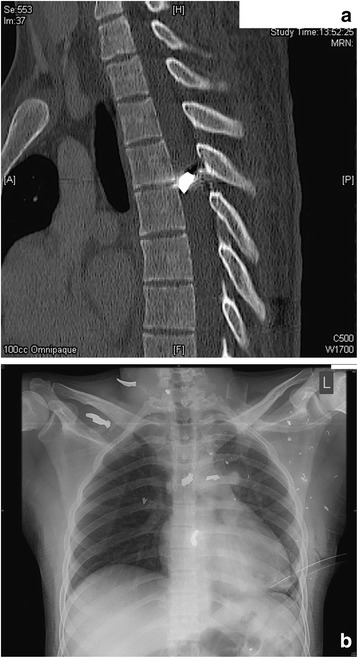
a Sagittal CT images showing the metallic foreign body within the spinal canal at the level of T5. b Plain chest radiograph showing multiple foreign bodies as typically seen in IED blast injuries
